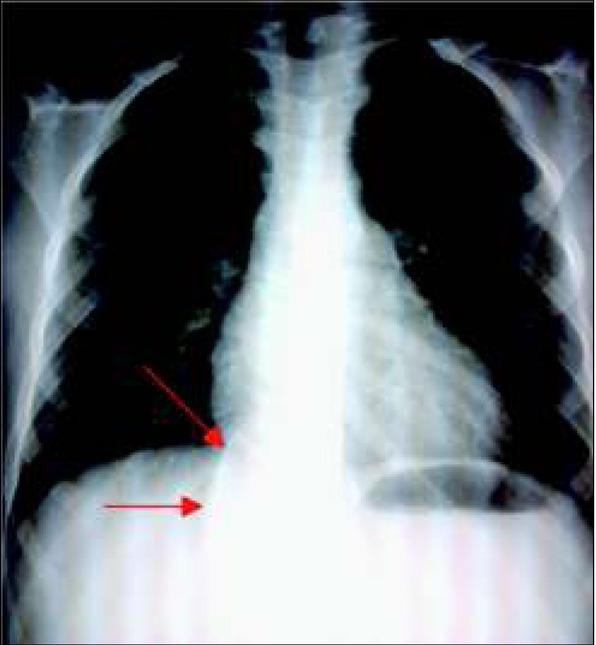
Zdjęcie RTG klatki piersiowej, w projekcji AP (strzałkami zaznaczono widoczny cień krągły) Fig. 1. A chest X-ray, anteroposterior view, showing a nodule (marked by arrows).
